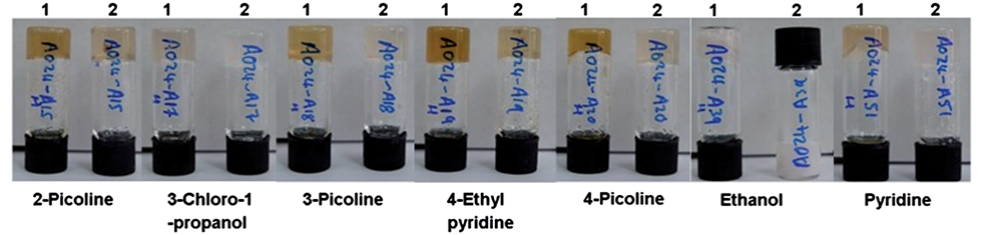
Comparison between gels of 1a formed by a combination of ultrasound and heat treatment (labelled 1) and room temperature sonogels (2) at 1 wt%.
